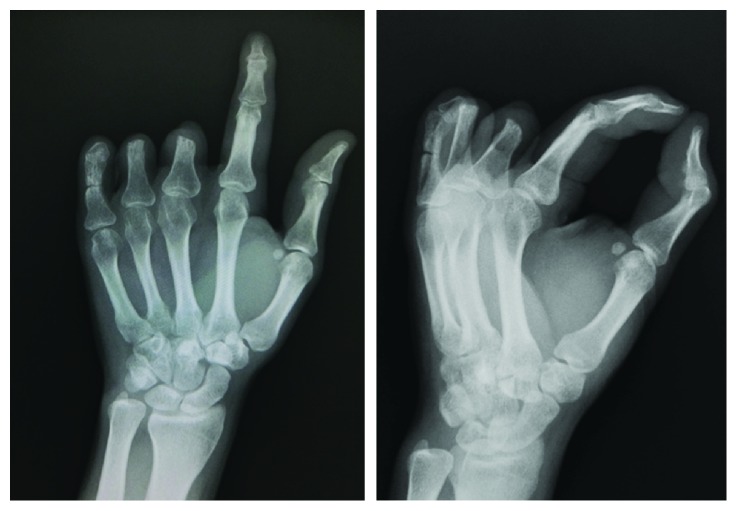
Preoperative X-ray confirms findings based on hand appearance. Nonunion of the proximal phalanx is seen.
